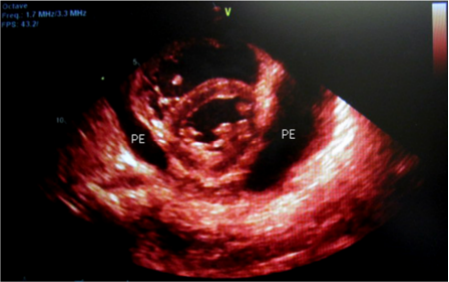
Two-dimensional transthoracic echocardiography visualized a large circumferential pericardial effusion (PE).
